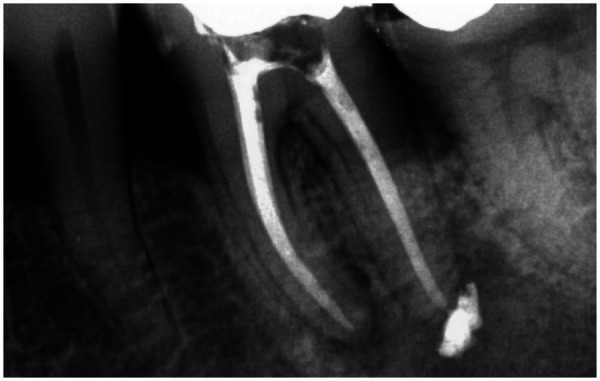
Post-obturation intraoral radiograph of tooth 36.
